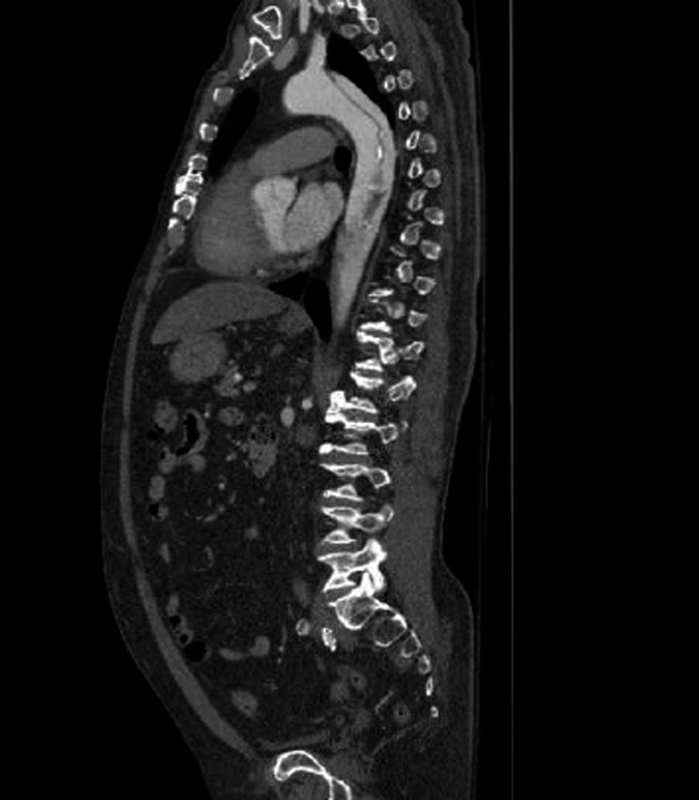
Computed tomography angiography showing the entry tear of the dissection just below the origin of the left subclavian artery.
